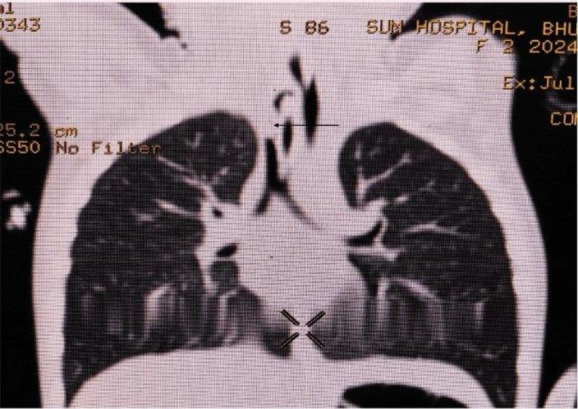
HRCT thorax shows a well-defined oval-shaped hypodense submucosal lesion of size 9.4x7.5x12. 5 mm arising from the right lateral wall of trachea, approximately 2.2 cm proximal to the carina (indicated by red arrow) with endotracheal tube in situ (indicated by green arrow)
